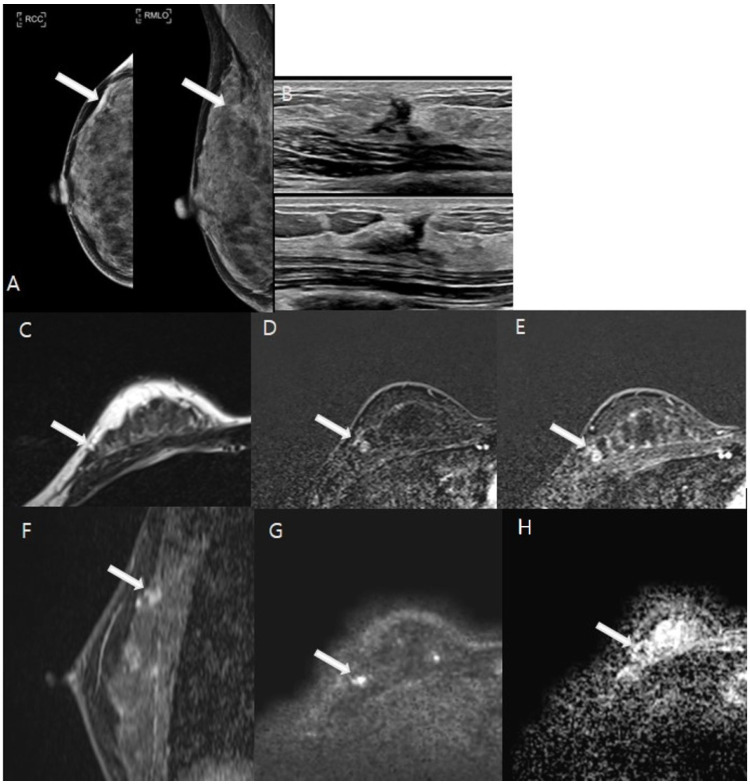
A 47-year-old woman underwent vacuum-assisted excisional biopsy and was diagnosed with ductal carcinoma in situ (DCIS). White arrows indicate the lesions in the following images. (A). Post-biopsy mammography showed architectural distortion with several round microcalcifications in the right upper outer quadrant. (B). Ultrasound revealed an irregular hypoechoic area corresponding to post-biopsy changes in the same region. (C–H). A 1 cm focal area of T2 isointense signal was observed adjacent to the post-biopsy changes (C). This area exhibited heterogeneous enhancement from the initial to the delayed phase on axial images (D,E), with enhanced visualization on sagittal images (F). Diffusion-weighted imaging (DWI) at b = 1000 mm^2^/s and the ADC map (b = 0 and 1000 mm^2^/s) indicated restricted diffusion within the lesion, with an ADC value of 0.9 × 10^−3^ mm^2^/s (G,H). The patient subsequently underwent breast-conserving surgery, which confirmed a residual 0.8 cm grade 1 DCIS on pathological examination.
